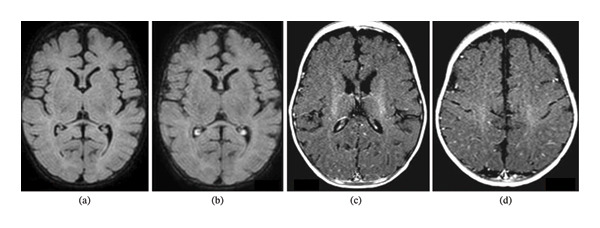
FLAIR sequence pre‐ (a) and post‐Gd (b) failed to attest to higher CSF signal intensity post‐Gd. T1w_Gd demonstrates increased supratentorial leptomeningeal enhancement, most prominently involving occipital regions bilaterally at the level of the superior aspects of the basal ganglia (c), as well as biparietal (d).
